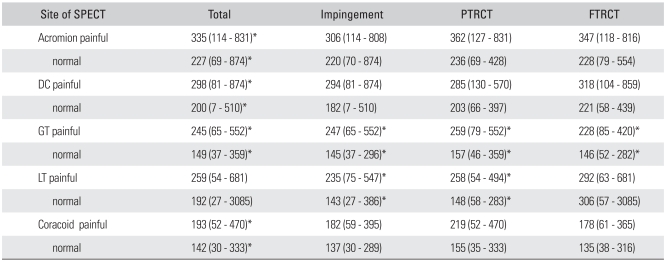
Average Scores and Ranges of Several Anatomic Sites in Painful Shoulder Using Quantitative SPECT
